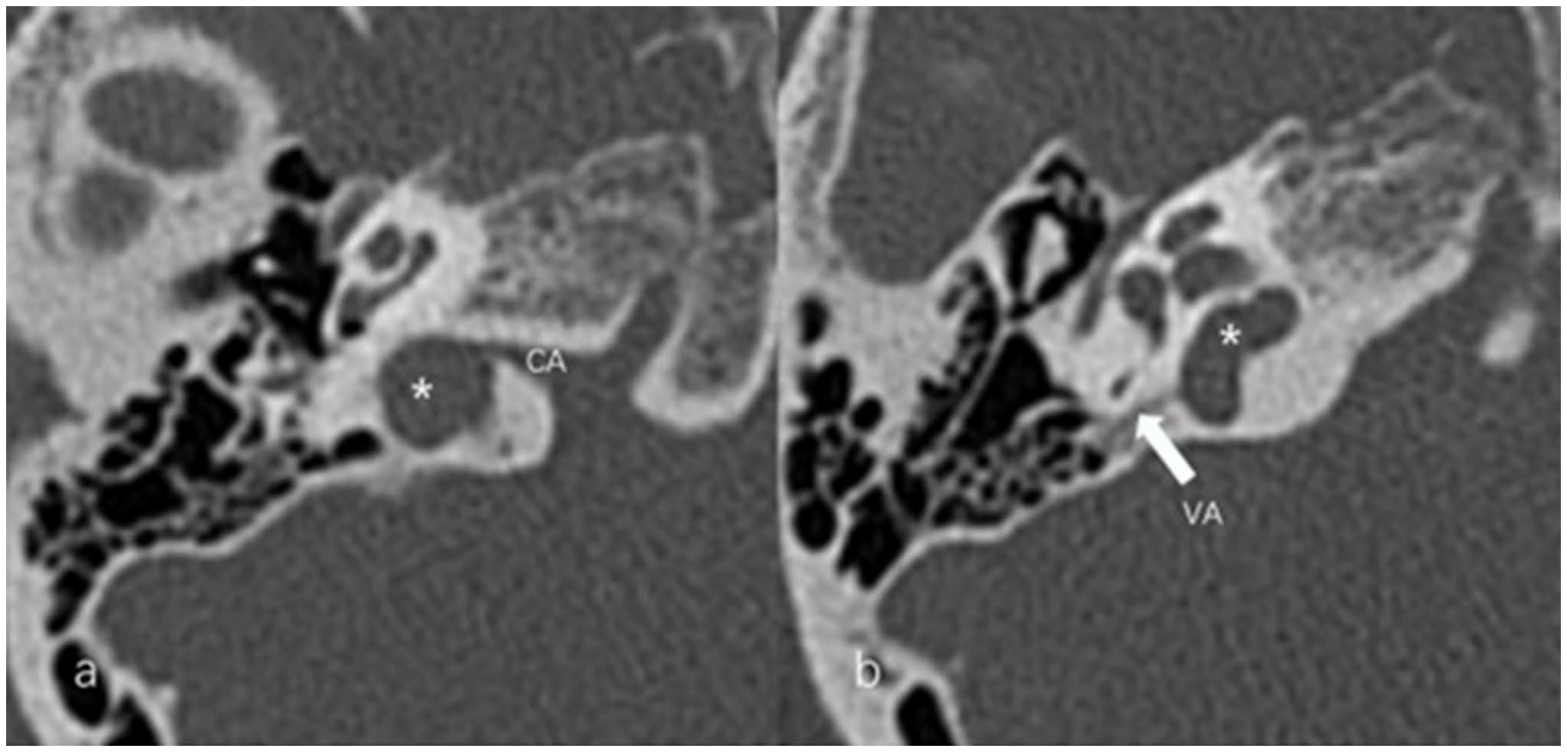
Jugular bulb related inner ear dehiscence (JBID). (a) Bony dehiscence between jugular bulb (JB) (asterisk) and the cochlear aqueduct (CA) on the axial image of temporal bone high-resolution computed tomography (HRCT). (b) Bony dehiscence between JB (asterisk) and the vestibular aqueduct (arrow) on the axial image of temporal bone HRCT.
